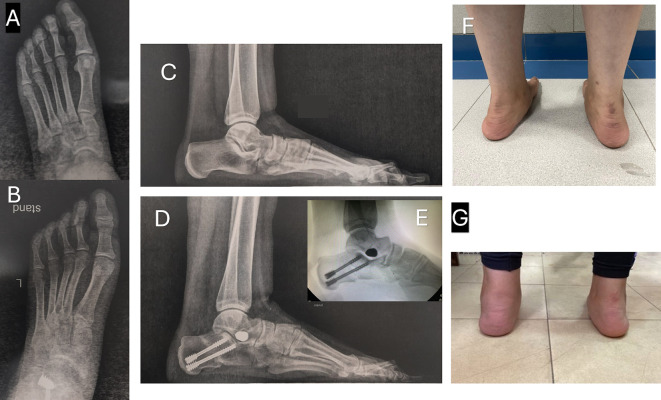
Pre- and post-operative X-rays and clinical photographs of 18-year-old female underwent left foot MDCO + STA. A: Preoperative A-P weight-bearing X-ray. B: Postoperative A-P weight-bearing X-ray. C: Preoperative lateral weight-bearing X-ray. D: Preoperative lateral weight-bearing X-ray at the last follow-up. E: Intraoperative X-ray demonstrates STA + MDCO technique. F: Preoperative clinical photo showing the left foot hindfoot valgus. G: Postoperative clinical photo with evidence left foot hindfoot valgus correction at the last follow-up.
